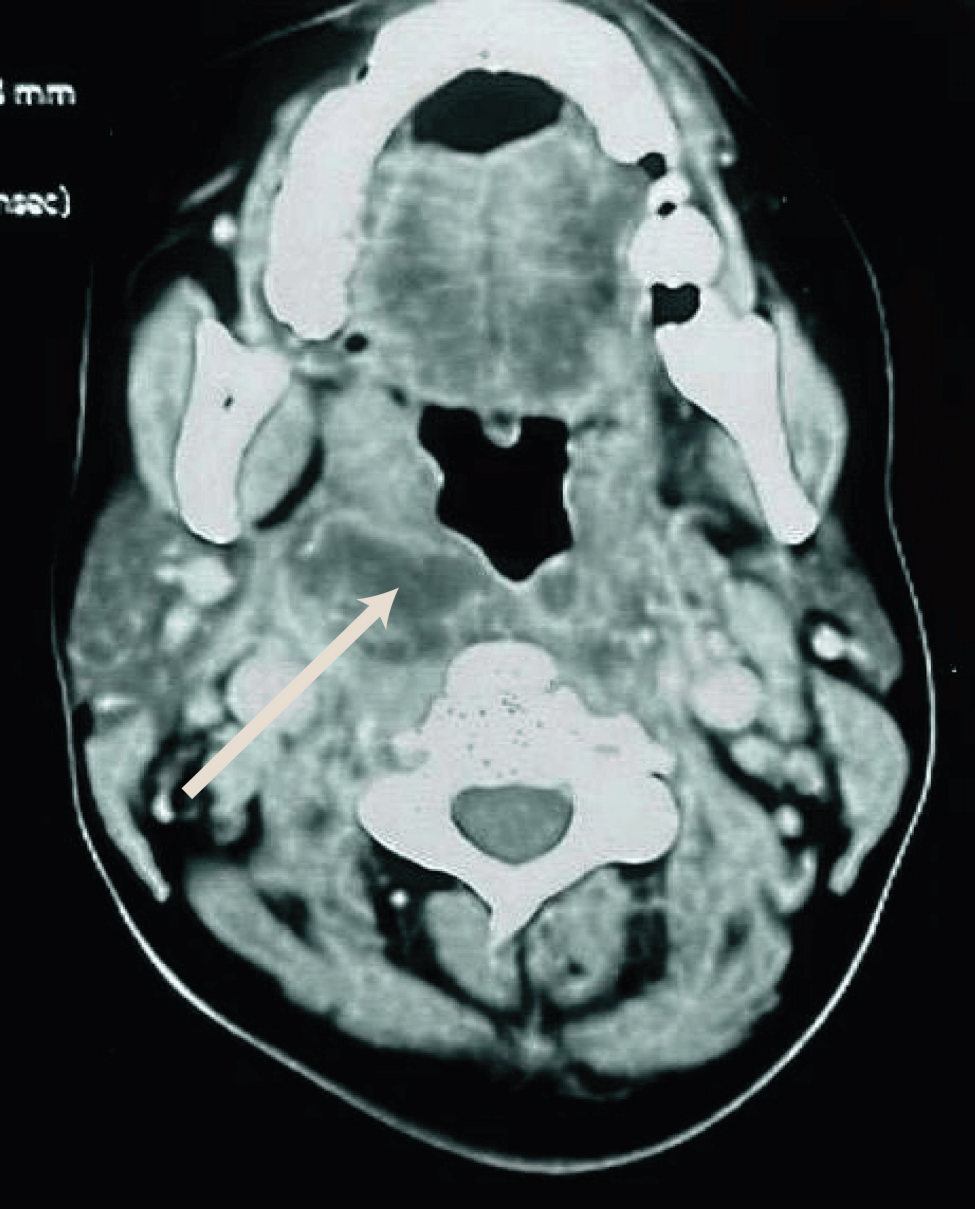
Cervical CT, axial view, parenchymal window, showing abscess collections in the retro- and parapharyngeal spaces
